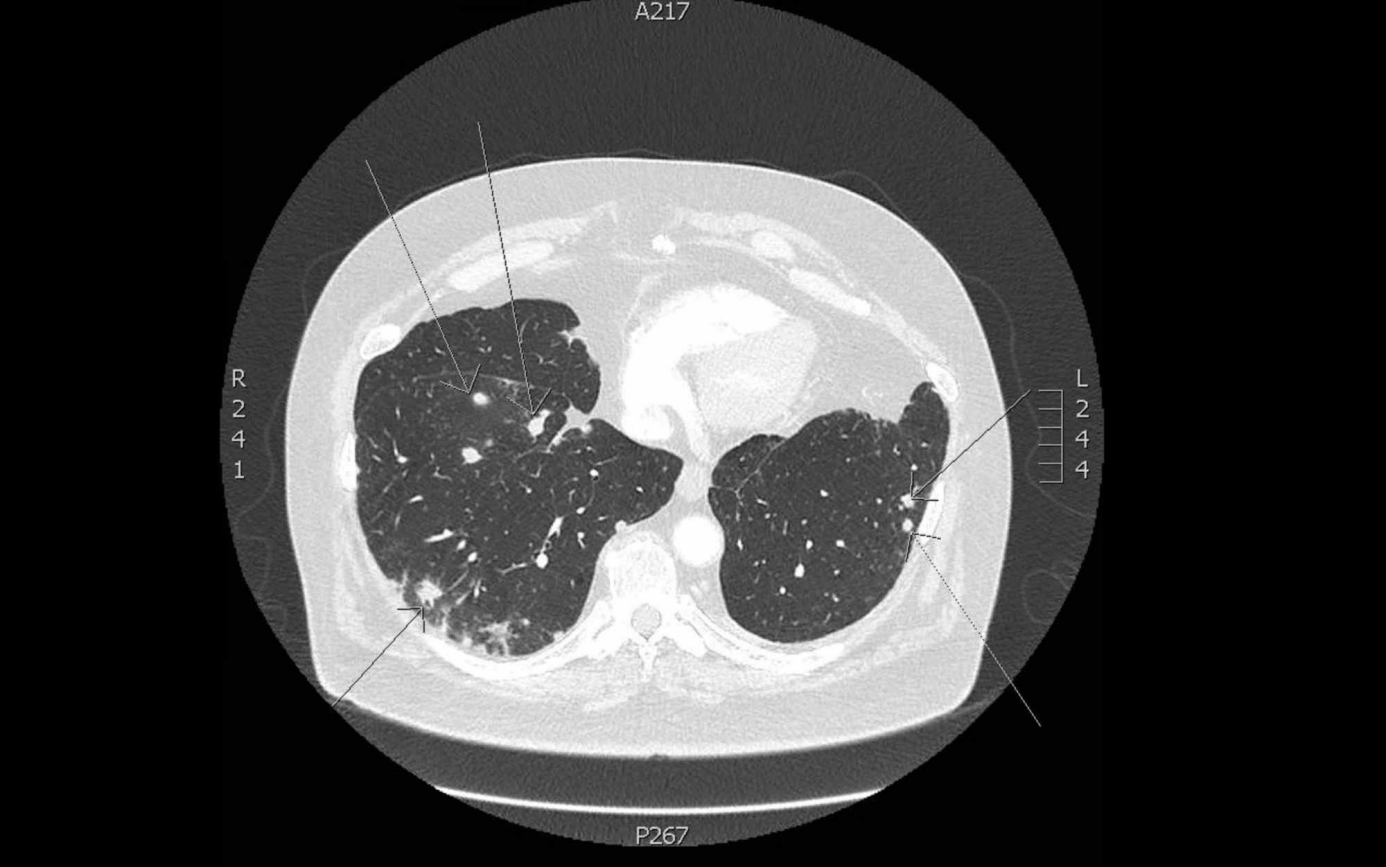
CT chest demonstrating numerous cavitary nodular opacities (arrows). CT: Computed tomography
